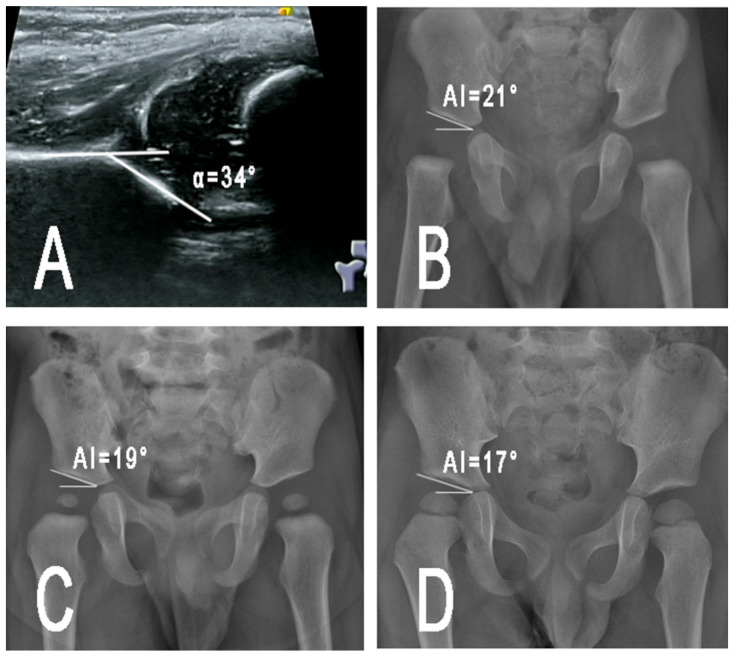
A patient aged 4 months with right DDH (Graf type III) (A), treated by PH for 4 months and recovered to normal radiograph (B). Six months later, radiographic examination showed good development of the hip (C). At final follow-up (2 years old), the outcome is satisfactory (D).
